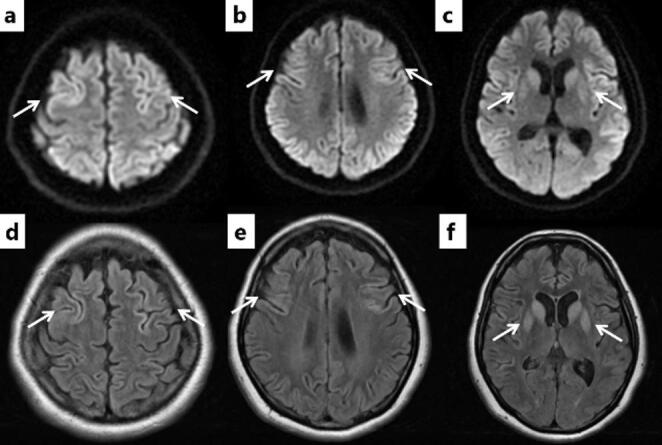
Hypoglycemic encephalopathy. a–c DWI, d–f FLAIR. A woman in her 30s with type 2 DM accidentally ingested a large sulfonylurea dose, resulting in prolonged loss of consciousness. DWI (a–c) and FLAIR (d–f) show bilateral symmetric hyperintensities in the frontal and parietal lobes and the striatum (arrow). This distribution differs from that of vascular territorial infarctions. Abbreviations: DM, diabetes mellitus; FLAIR, fluid-attenuated inversion recovery; DWI, diffusion-weighted imaging
